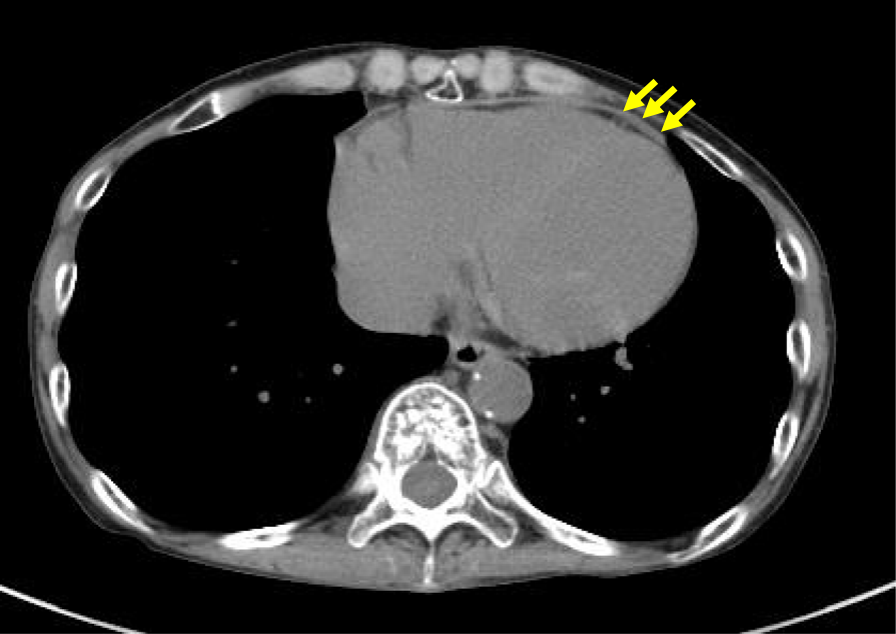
Computed tomography showed moderate pericardial thickening. (yellow arrow)
